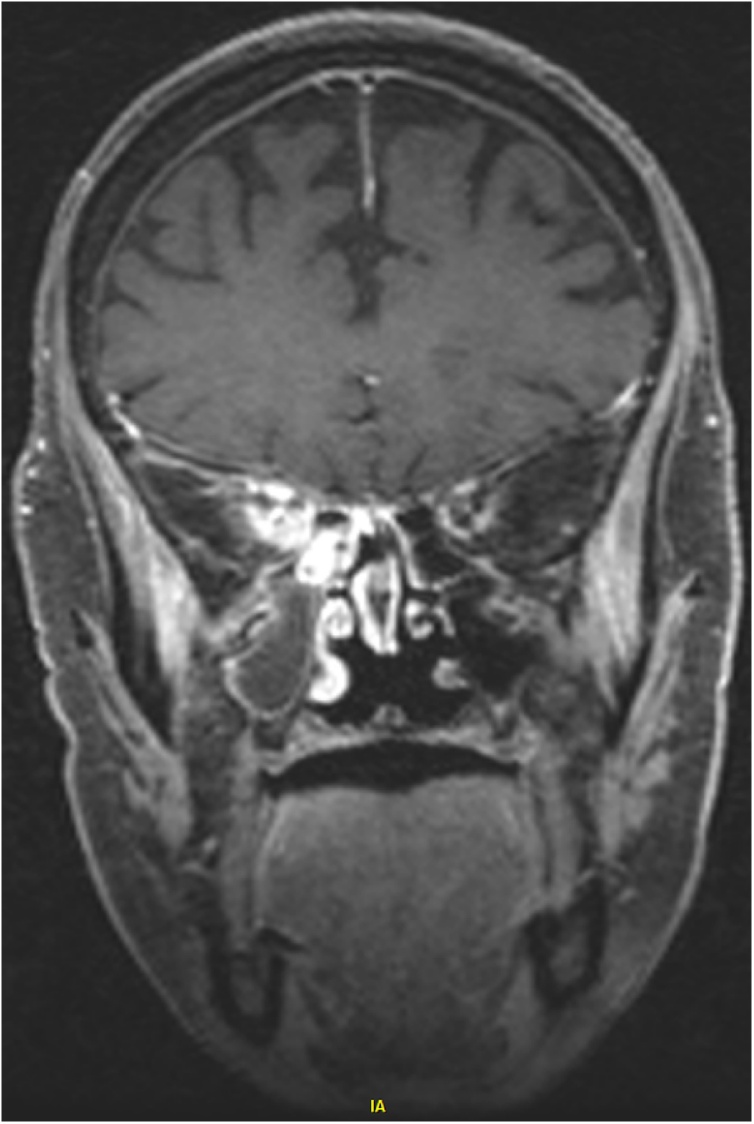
MRI of the brain, coronal view postcontrast T1 fat-suppressed CUBE, demonstrating phlegmonous extension of the right paranasal sinus infection into the orbital apex and toward the right cavernous sinus.
